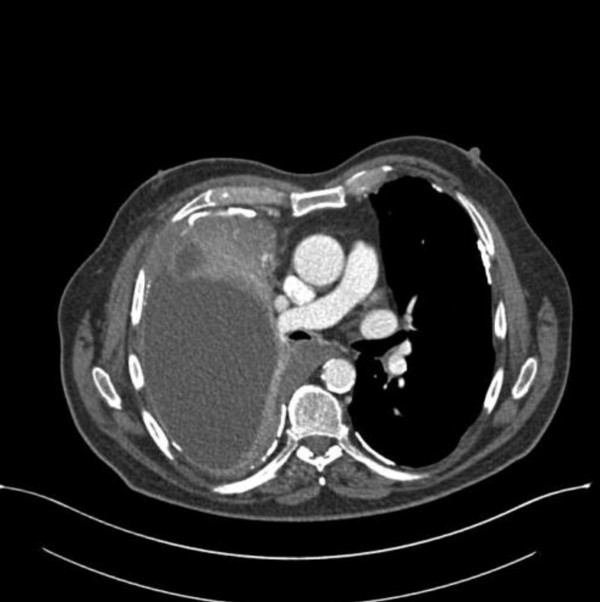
Chest CT presenting the mesothelioma
